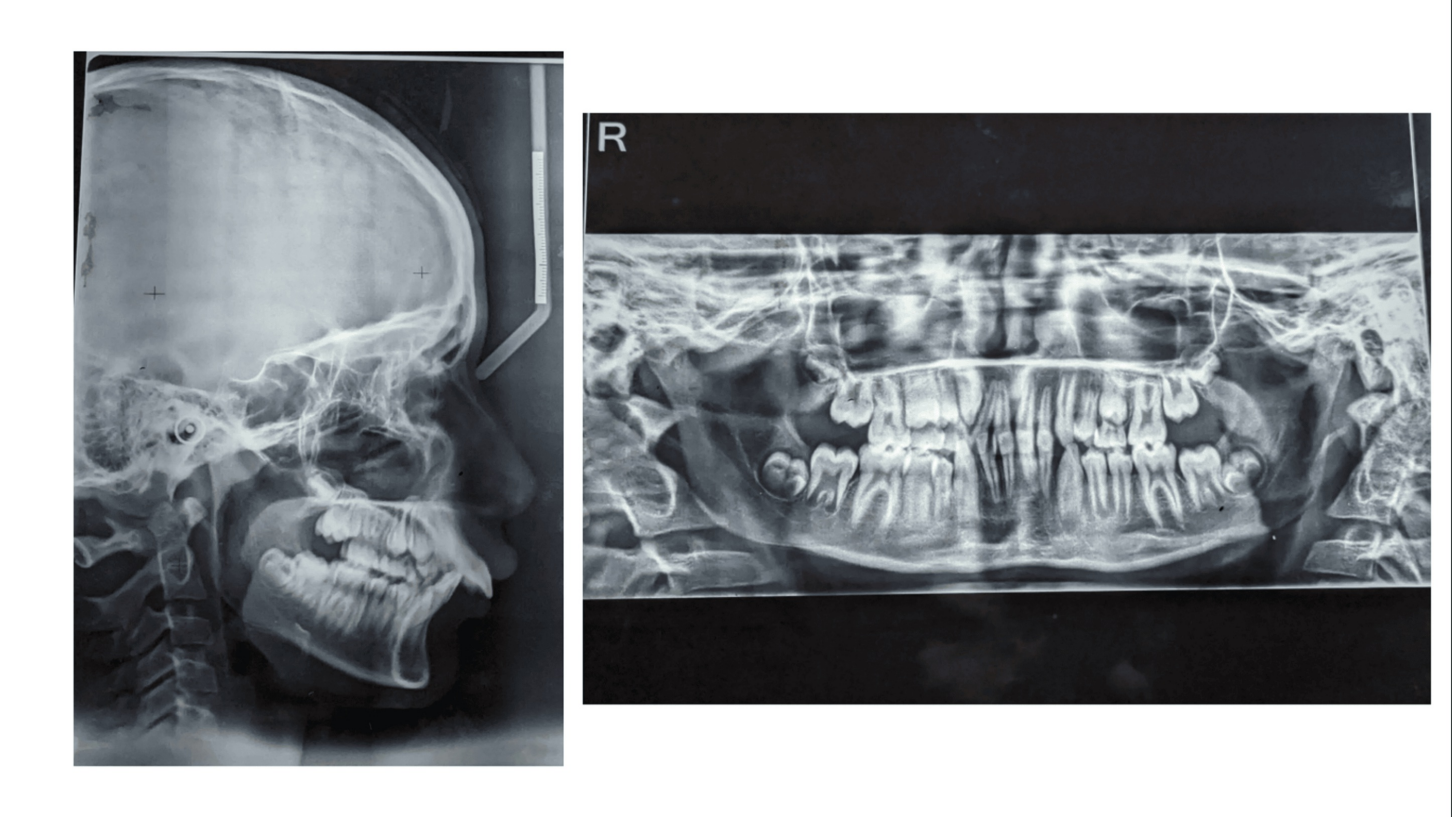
Pretreatment lateral cephalogram and orthopantomogram
